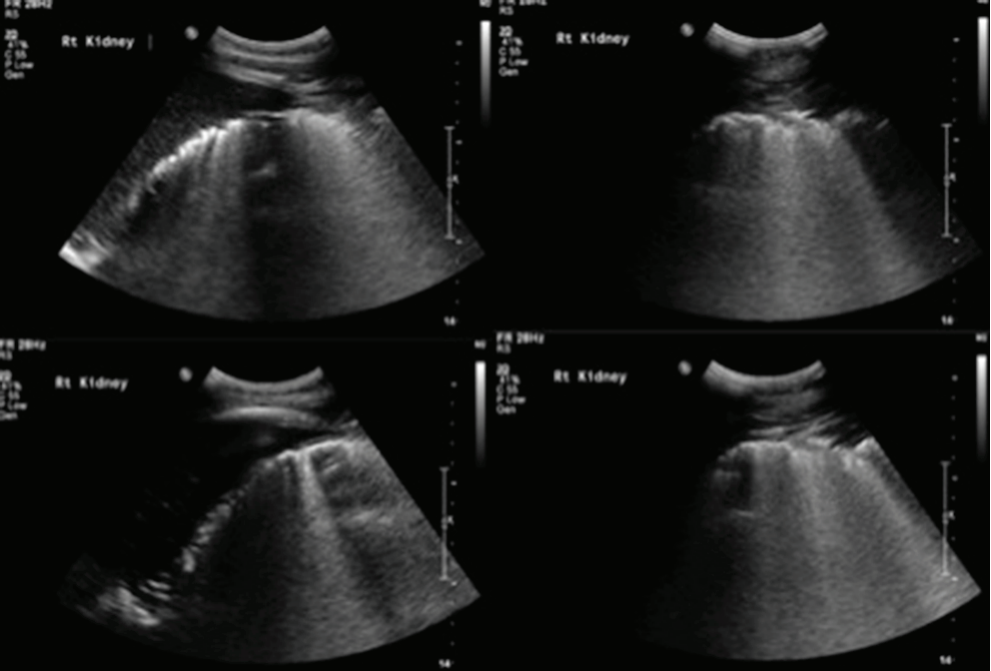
Ultrasound images of right kidney demonstrating the difficulty in obtaining clear images.
